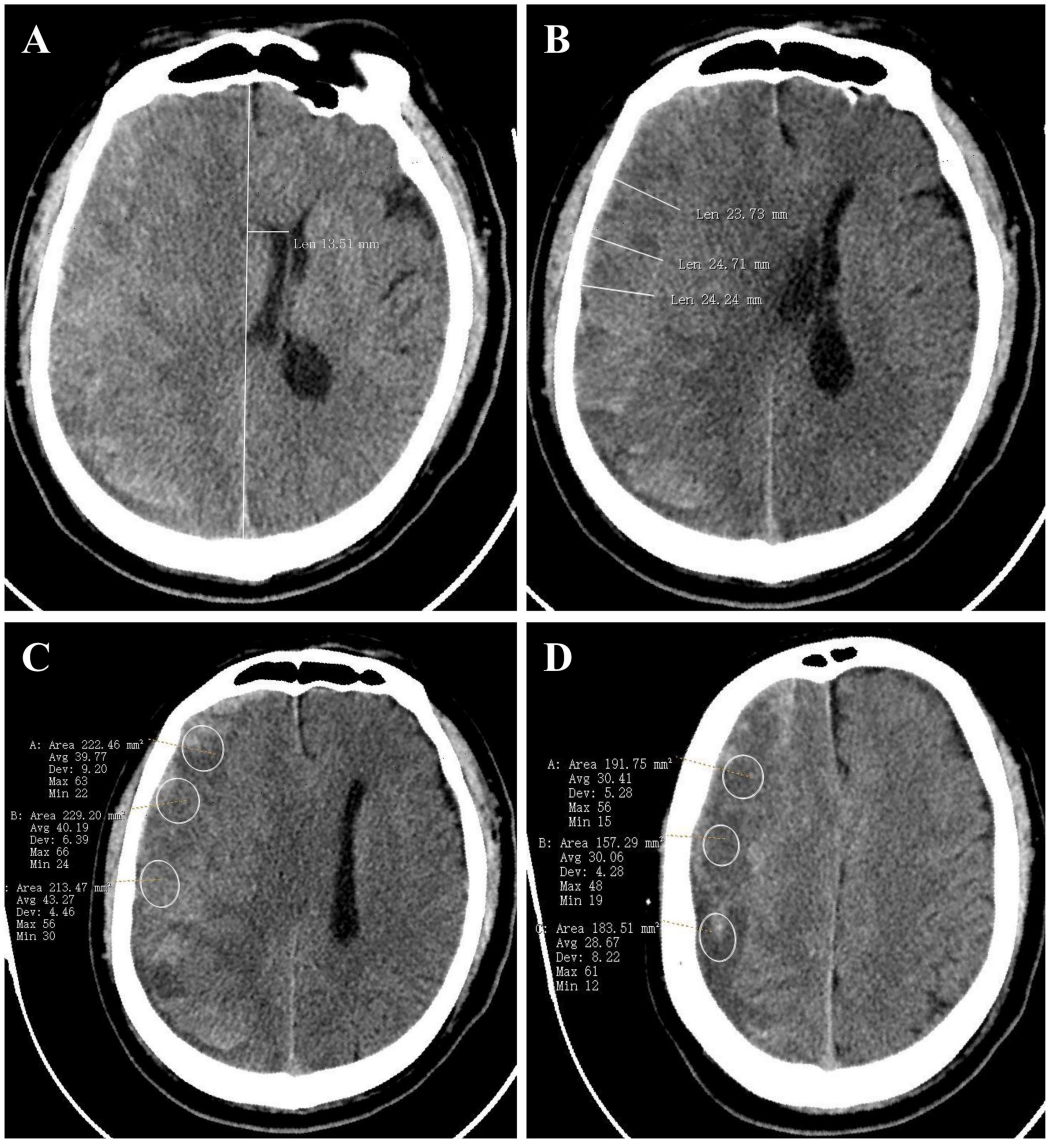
The data measurement method on CT. (A–D) show the measurement method of MS (A), HT (B), and hematoma density (C,D), separately. MS, midline shift; HT, maximal hematoma thickness.
